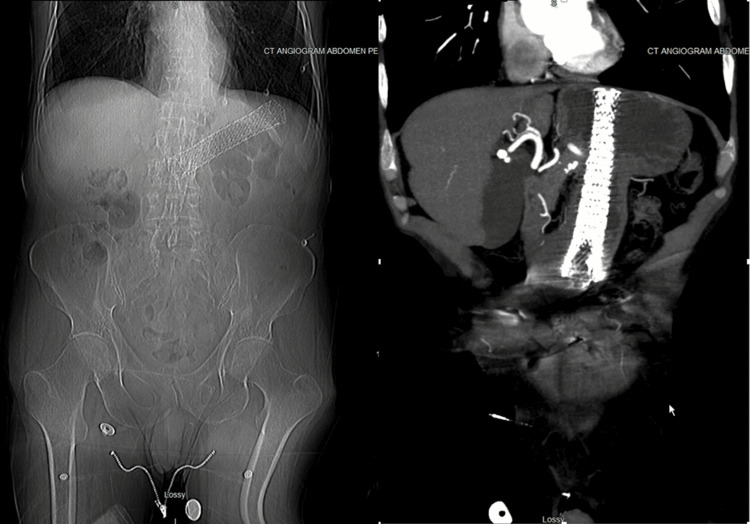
CT scout image and CT angiogram of abdomen and pelvis with malpositioned esophageal stent within the stomach.
